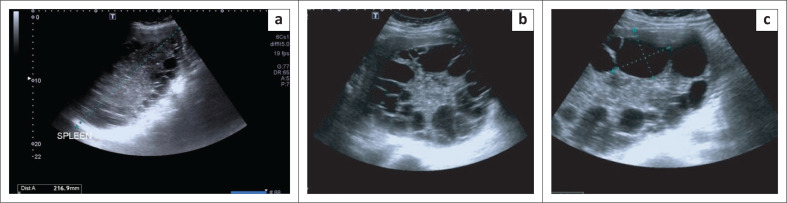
Images taken from the initial ultrasound done on presentation; (a) An image showing the enlarged spleen measuring 21 cm; (b) This image shows the extensive splenic hydatid cysts involving more than 90% of the normal splenic tissue; (c) An indication of the largest splenic cyst measuring 4.8 cm × 4.0 cm.
